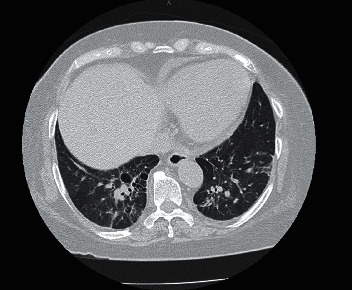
CT chest showing fibrotic parenchyma of both lungs more on the right side with nodules.
